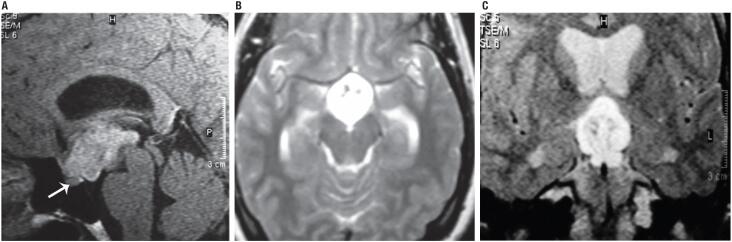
Patient 2. Magnetic resonance imaging findings: sagittal and coronal images. A suprasellar mass with heterogeneous enhancement after gadolinium administration is shown, with hypothalamic involvement and hydrocephalus. (A) The sagittal T1 weighted image shows a suprasellar tumor with gadolinium enhancement compromising the interpeduncular cistern and the third ventricle. The normal pituitary gland is in a normal position (arrow). (B) The axial T2 weighted image shows spontaneous hyperintensity. (C) The coronal FLAIR shows tumor brightness and associated obstructive hydrocephalus.
